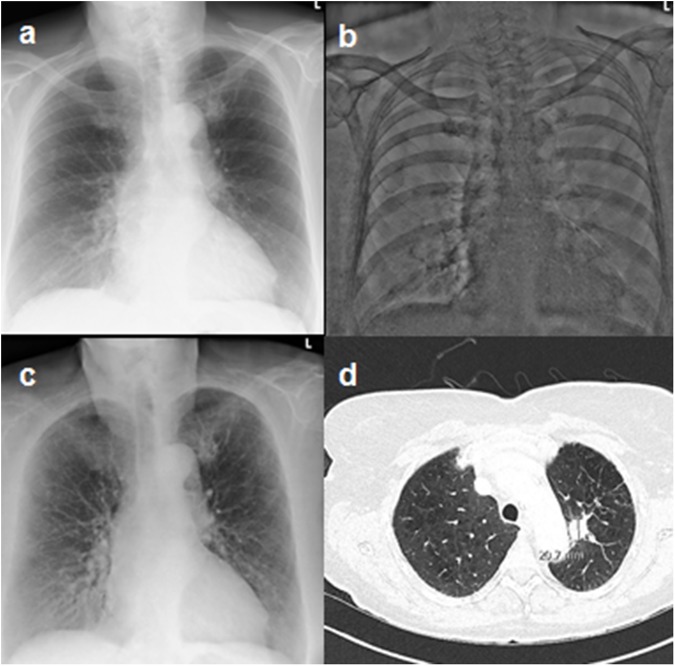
Lung mass on different imaging modalities. 61 year old female patient showing a focal increase in density in the left upper lobe in the CR image (a). This focal increase in density is not visible on the bone image (b) while it is clearly depicted by the soft tissue image (c) and therefore is primarily suspicious for a soft tissue mass in the left upper lobe. CT (d) confirms the presence of a soft tissue mass in the left upper lobe adjacent to the aortic arch suspicious for a bronchus carcinoma.
